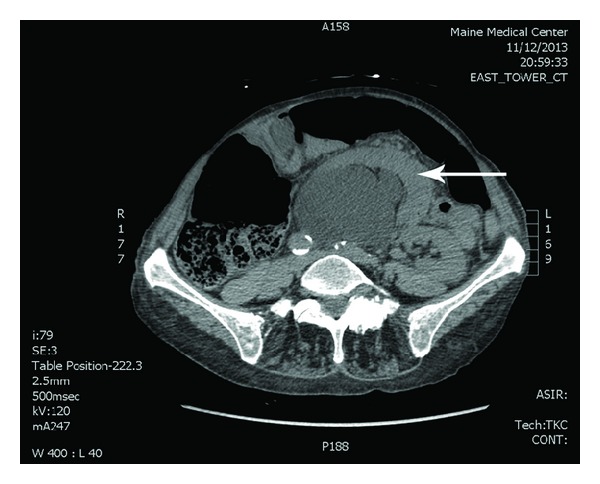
CT scan from November 2013 performed in the emergency department depicting right kidney (indicated by arrow) anterior to left kidney with extreme hydronephrosis.
